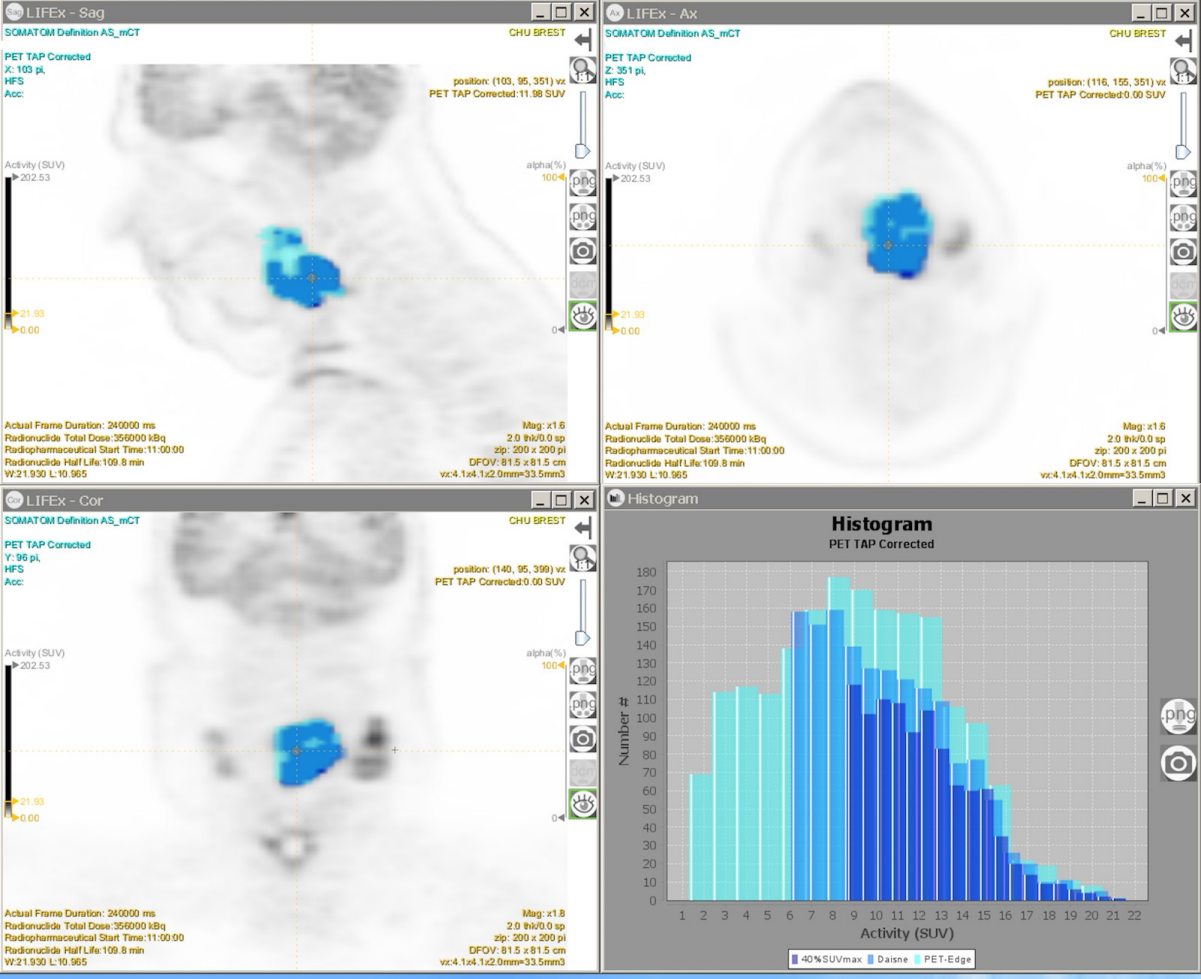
Example of VOI delineating a tumor with the 3 segmentation methods. (Turquoise blue) PET-EDGE segmentation method. (Sky blue) DAISNE segmentation method. (Dark blue) 40%SUVmax segmentation method. (Top left) FDG PET sagittal slice. (Top right) FDG PET transverse slice. (Bottom left) FDG PET frontal slice. (Bottom right) SUV histograms. With LIFEx software.
